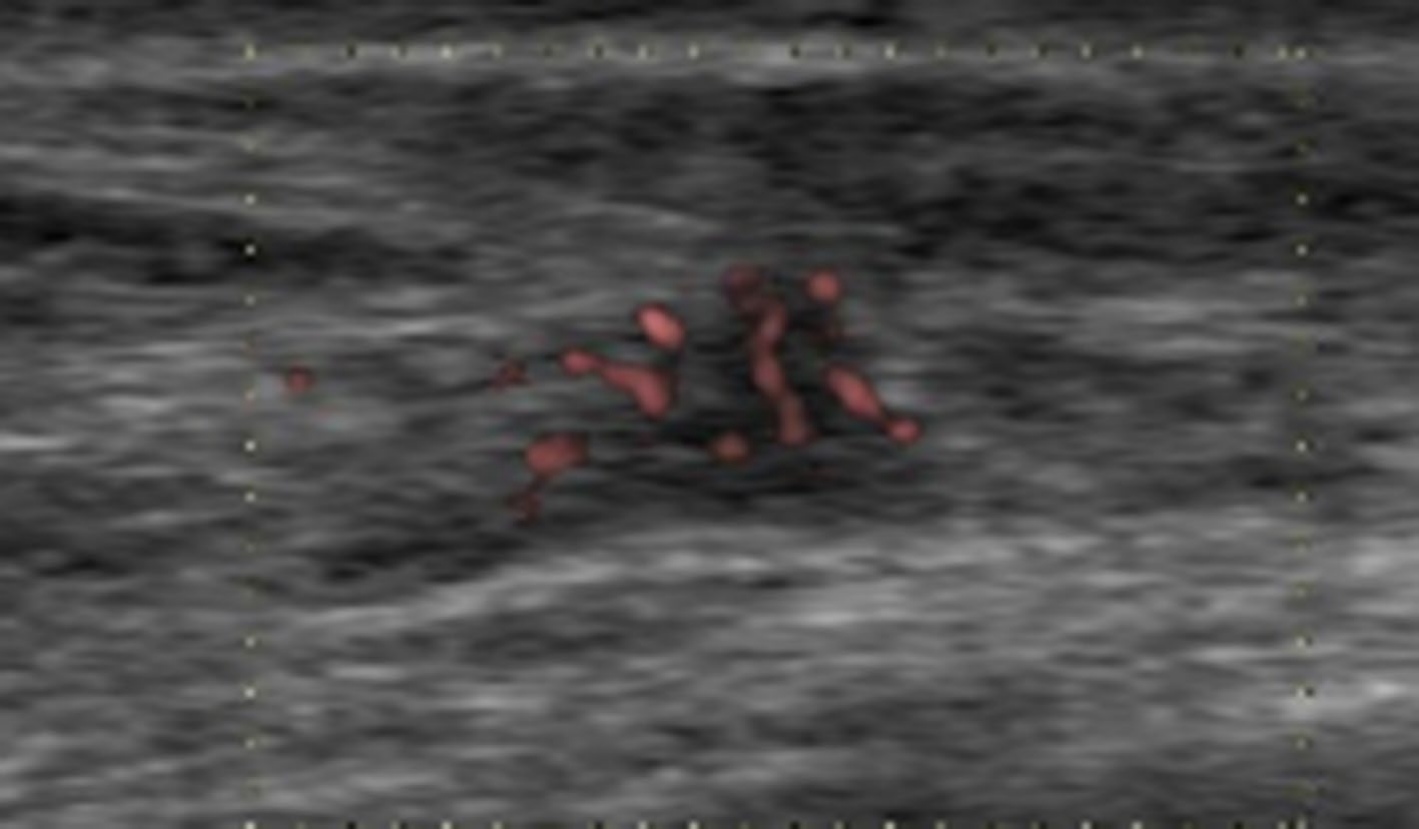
The power Doppler US image shows a non-mass lesion with some internal vascularity in a 76-year-old woman. A subsequent pathological examination confirmed intermediate-grade DCIS
